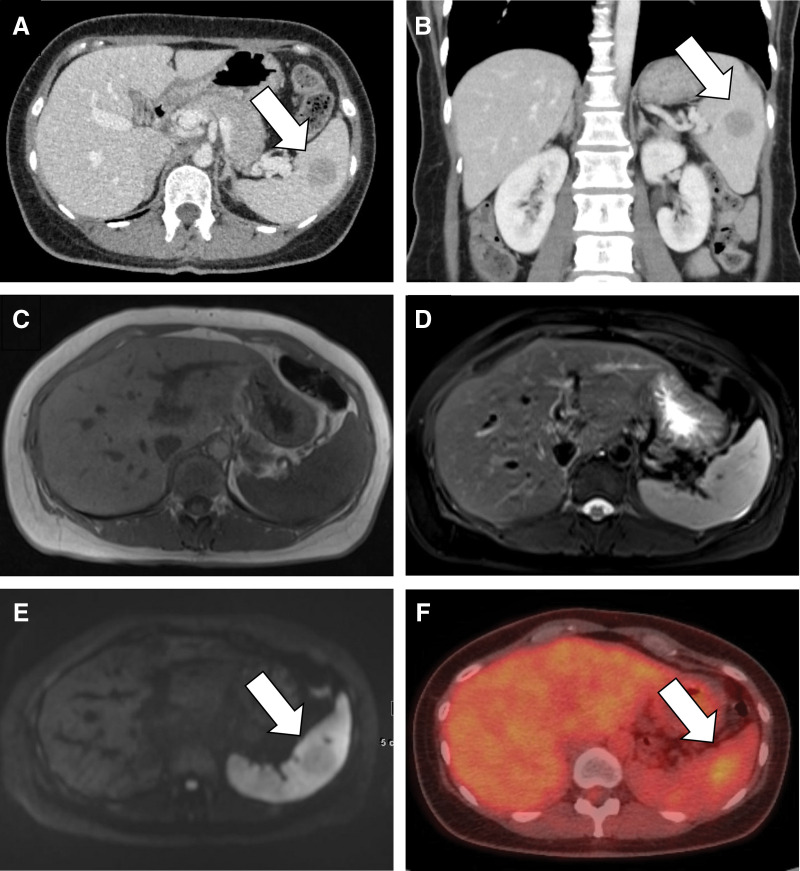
Imaging findings of Case 1. (A and B) In the venous phase, a 25-mm large round tumor in the spleen, which is lower than the adjacent splenic parenchyma on axial and coronal sections (white arrow), is enhanced on contrast-enhanced CT. (C) The tumor is not detected on T1-weighted images of the spleen on MRI. (D) The tumor is not detected on T2-weighted images of the spleen on MRI. (E) The tumor has a low signal compared to the splenic parenchyma on diffusion-weighted images in the MRI (white arrow). (F) PET/CT shows moderate uptake, with SUVmax = 3.0, in the spleen (white arrow). MRI T1/T2 iso-signal with the spleen is seen in SANT, but is also seen in malignant lymphoma and hamartoma. No “spoke-wheel pattern” is indicated on CT. Moderate uptake on PET/CT is observed in SANT. CT, computed tomography; MRI, magnetic resonance imaging; PET/CT, positron emission tomography/CT; SANT, sclerosing angiomatoid nodular transformation
